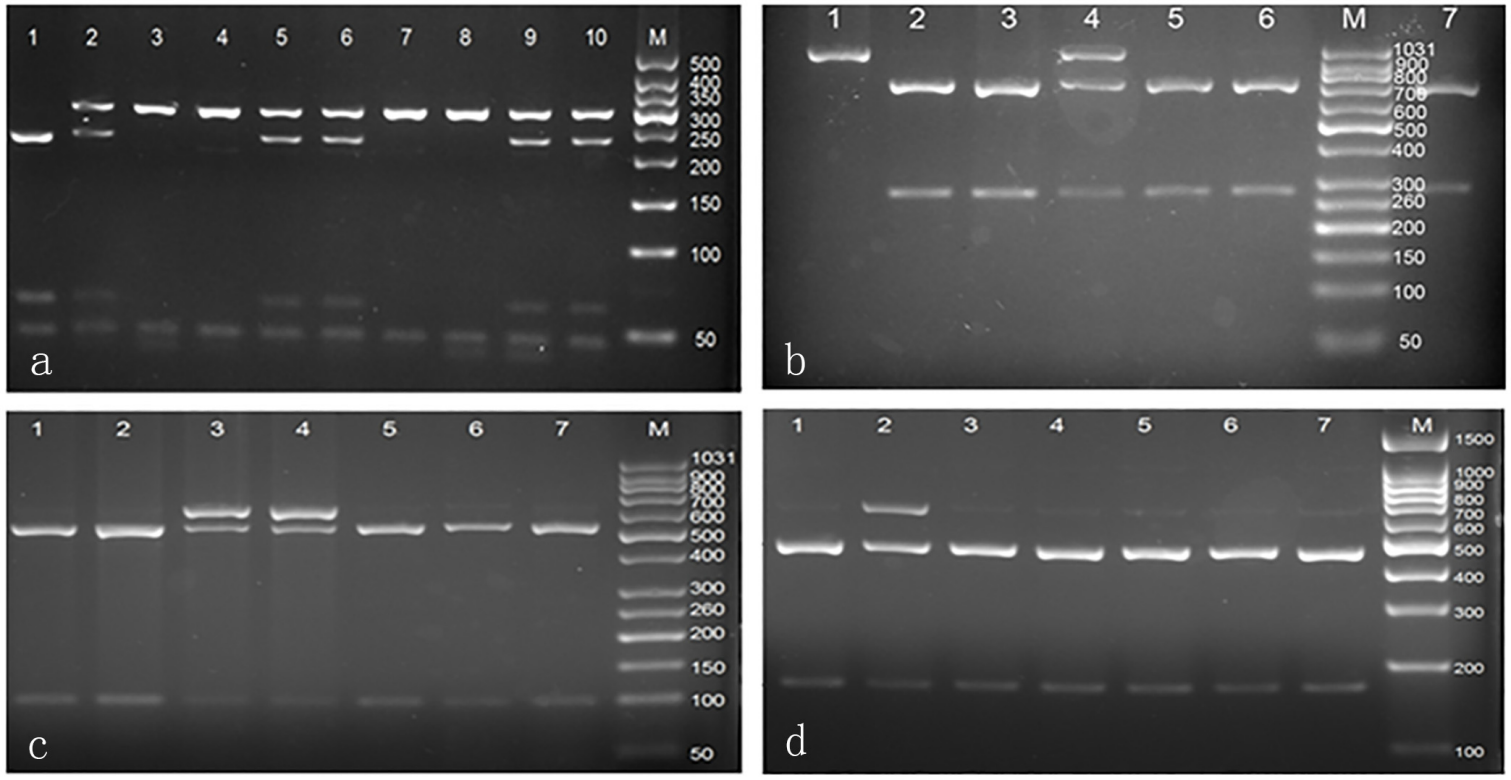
PCR-RFLP assay for analyzing the rs26311, rs27647, rs696217, and rs34911341 polymorphisms in GHRL gene. (a) rs26311—lane M shows DNA marker; lane 1 shows GG genotype; lanes 2, 5, 6, 9, and 10 show GC genotype; lanes 3, 4, 7, and 8 show CC genotype. (b) rs27647—lane M shows DNA marker; lanes 2, 3, 5, 6, and 7 show TT genotype; lane 4 shows TC genotype; lane 1 shows CC genotype. (c) rs696217—lane M shows DNA marker; lanes 1, 2, 5, 6, and 7 show GG genotype; lanes 3 and 4 show GT genotype. (d) rs34911341—lane M shows DNA marker; lanes 1, 3, 4, 5, 6, and 7 show CC genotype; lane 2 show CT genotype.
